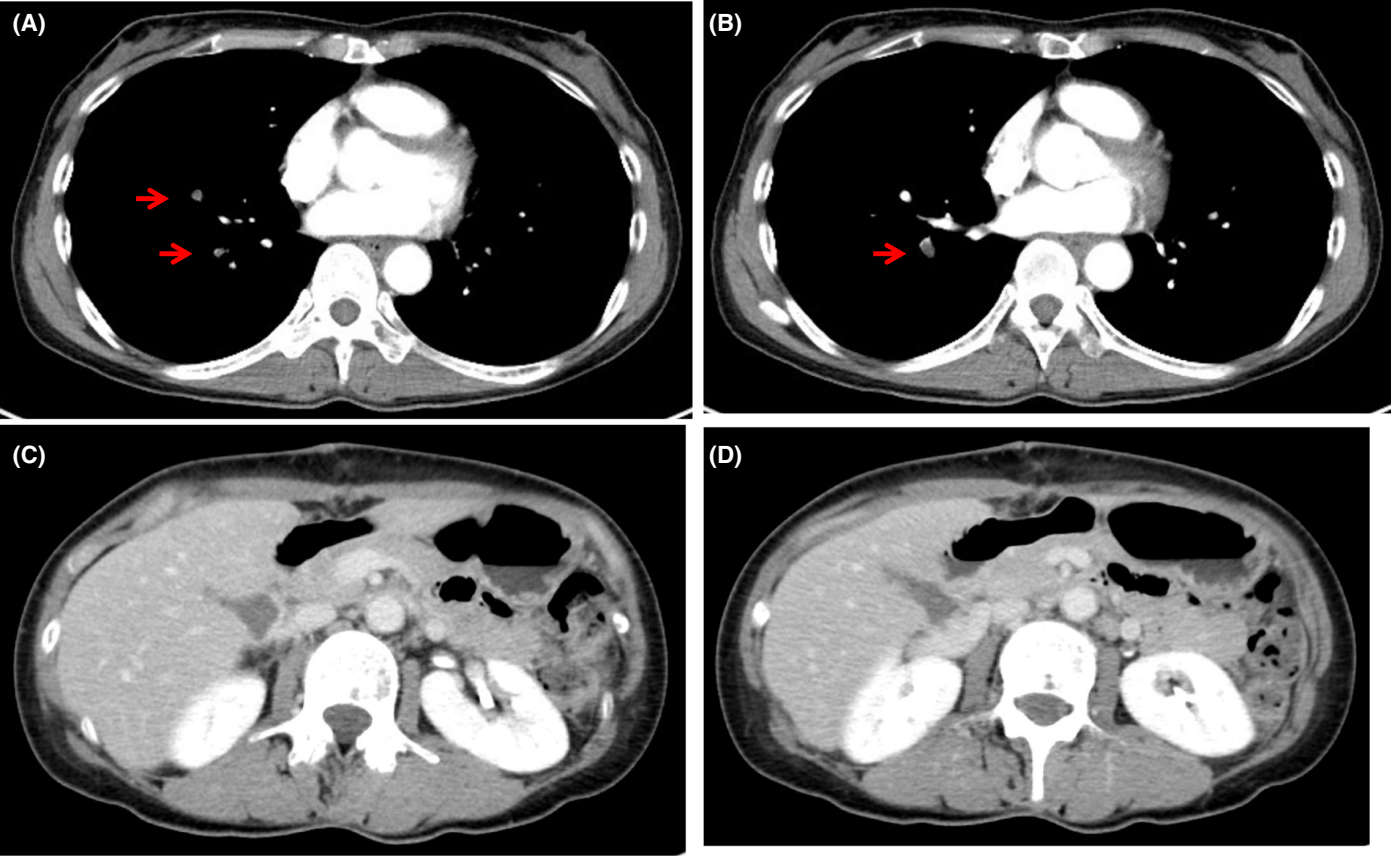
(A, B) On day 7 after the operation, CT revealed thrombosis of the peripheral pulmonary artery(A,B) but did not sow IVC thrombosis(C,D).
